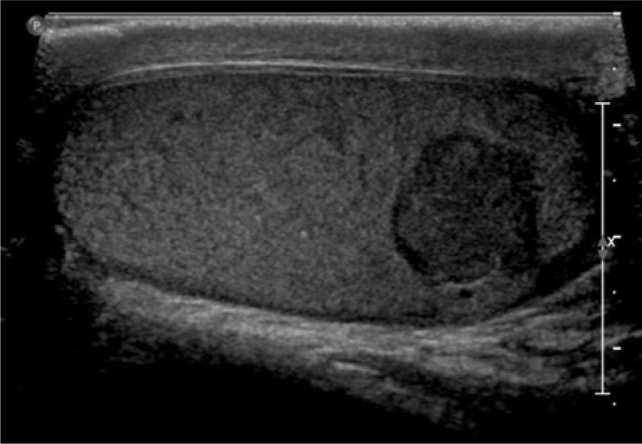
Ultrasound image showing 1.4 × 1.5 × 1.5 cm hypoechoic mass in the lower pole of right testicle.
